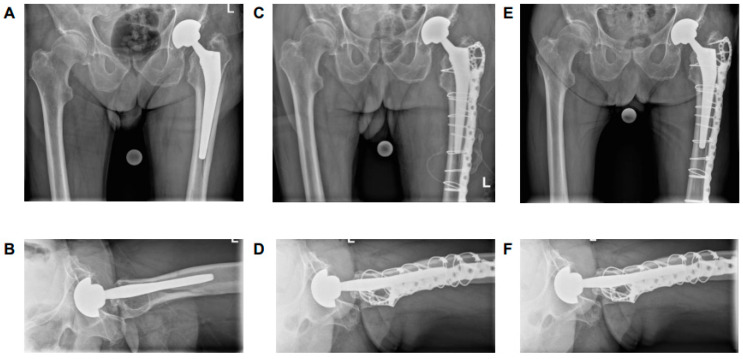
Antero-posterior and axial X-rays. (A,B) preoperatively; C-D postoperatively; E-F 12-week-follow-up. A 89-year-old men with PPF, left, sintered stem (18 mm) preoperatively (A,B), nearly anatomical reduction and fixation of the PPF during surgery (C,D), 12-week FU sintered (17 mm) to preoperative level (E,F) with a poor outcome including weakness and thigh pain.
